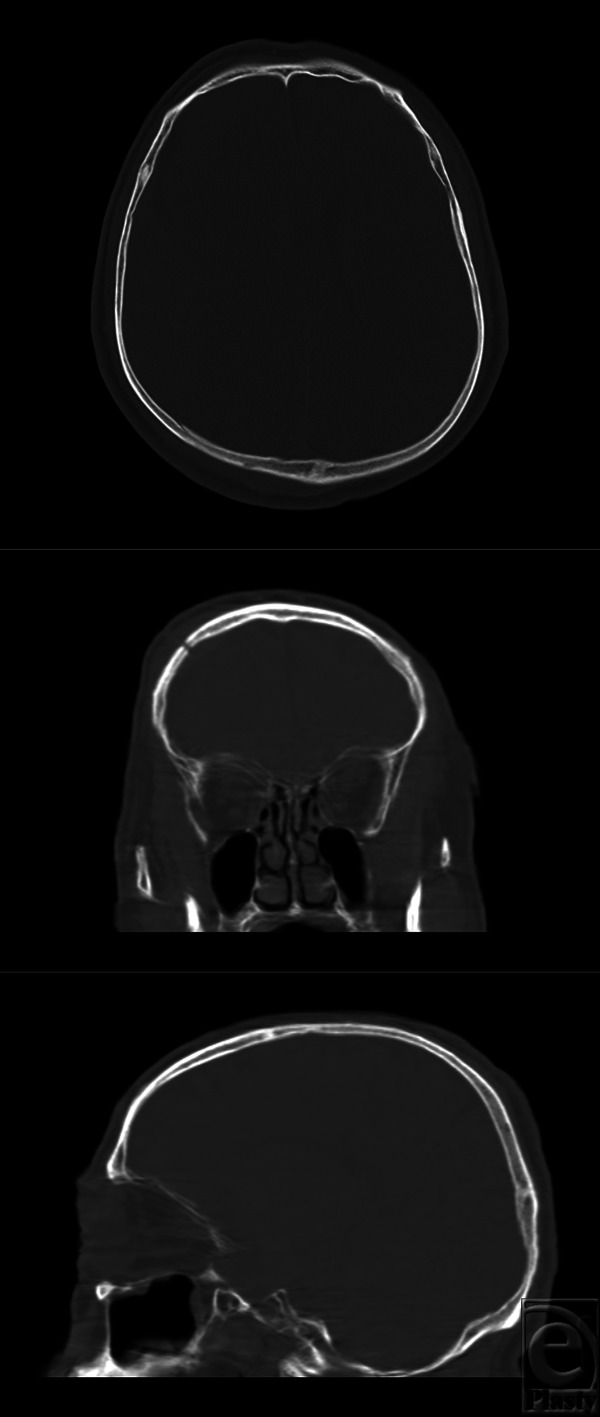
Initial brain computed tomography at the local hospital. Upper: axial view. Middle: coronal view. Lower: sagittal view. No definite fracture is seen on the left orbital roof. Parietal bone fractures are seen in the sagittal view.
